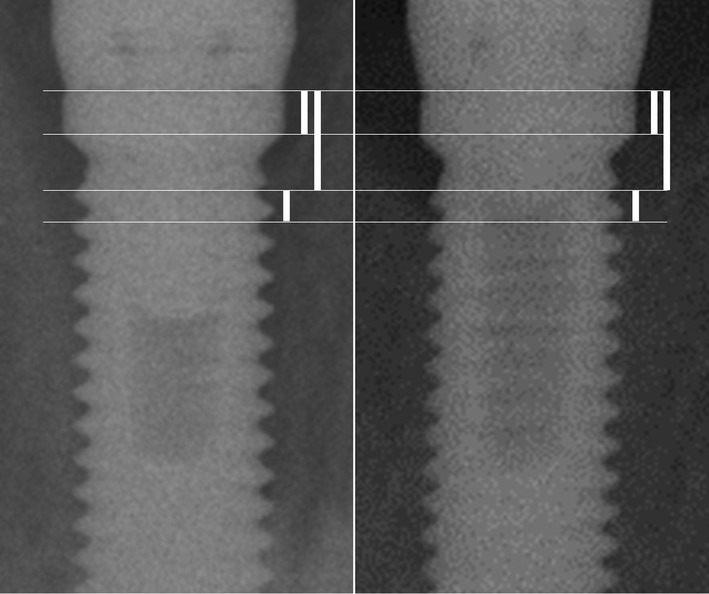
Details of radiographic bone measurement points using a Brånemark System Implant with a regular platform (ø = 3.75 mm). Vertical bars from top of shoulder to horizontal part of the shoulder = 0.8 mm; First thread = 1.8 mm; Second thread = 2.4 mm; Third thread = 3.0 mm etc. The interthread distances = 0.6 mm. Other dimensions apply for the narrow and wide platform implants.
